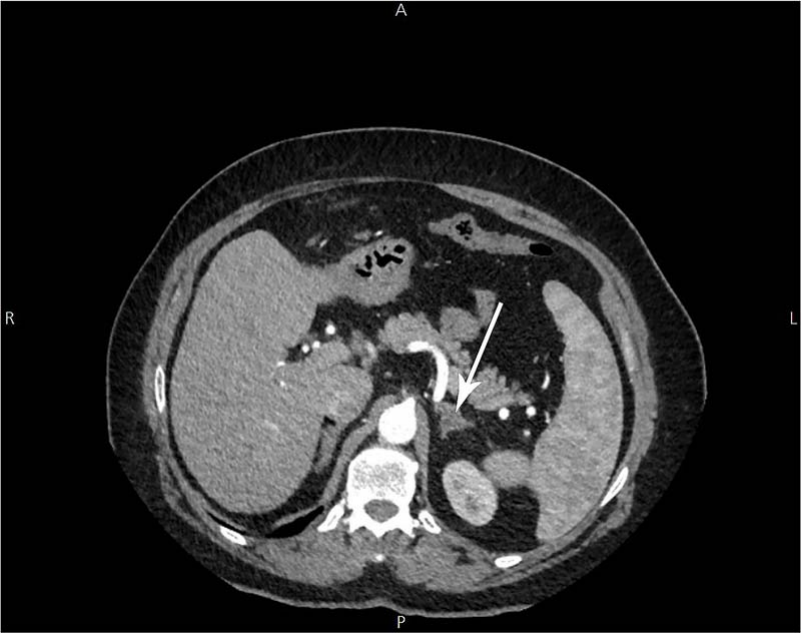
Arrow: a 1.5 cm non-enhancing hypodense nodule of the left suprarenal gland on CT imaging.
